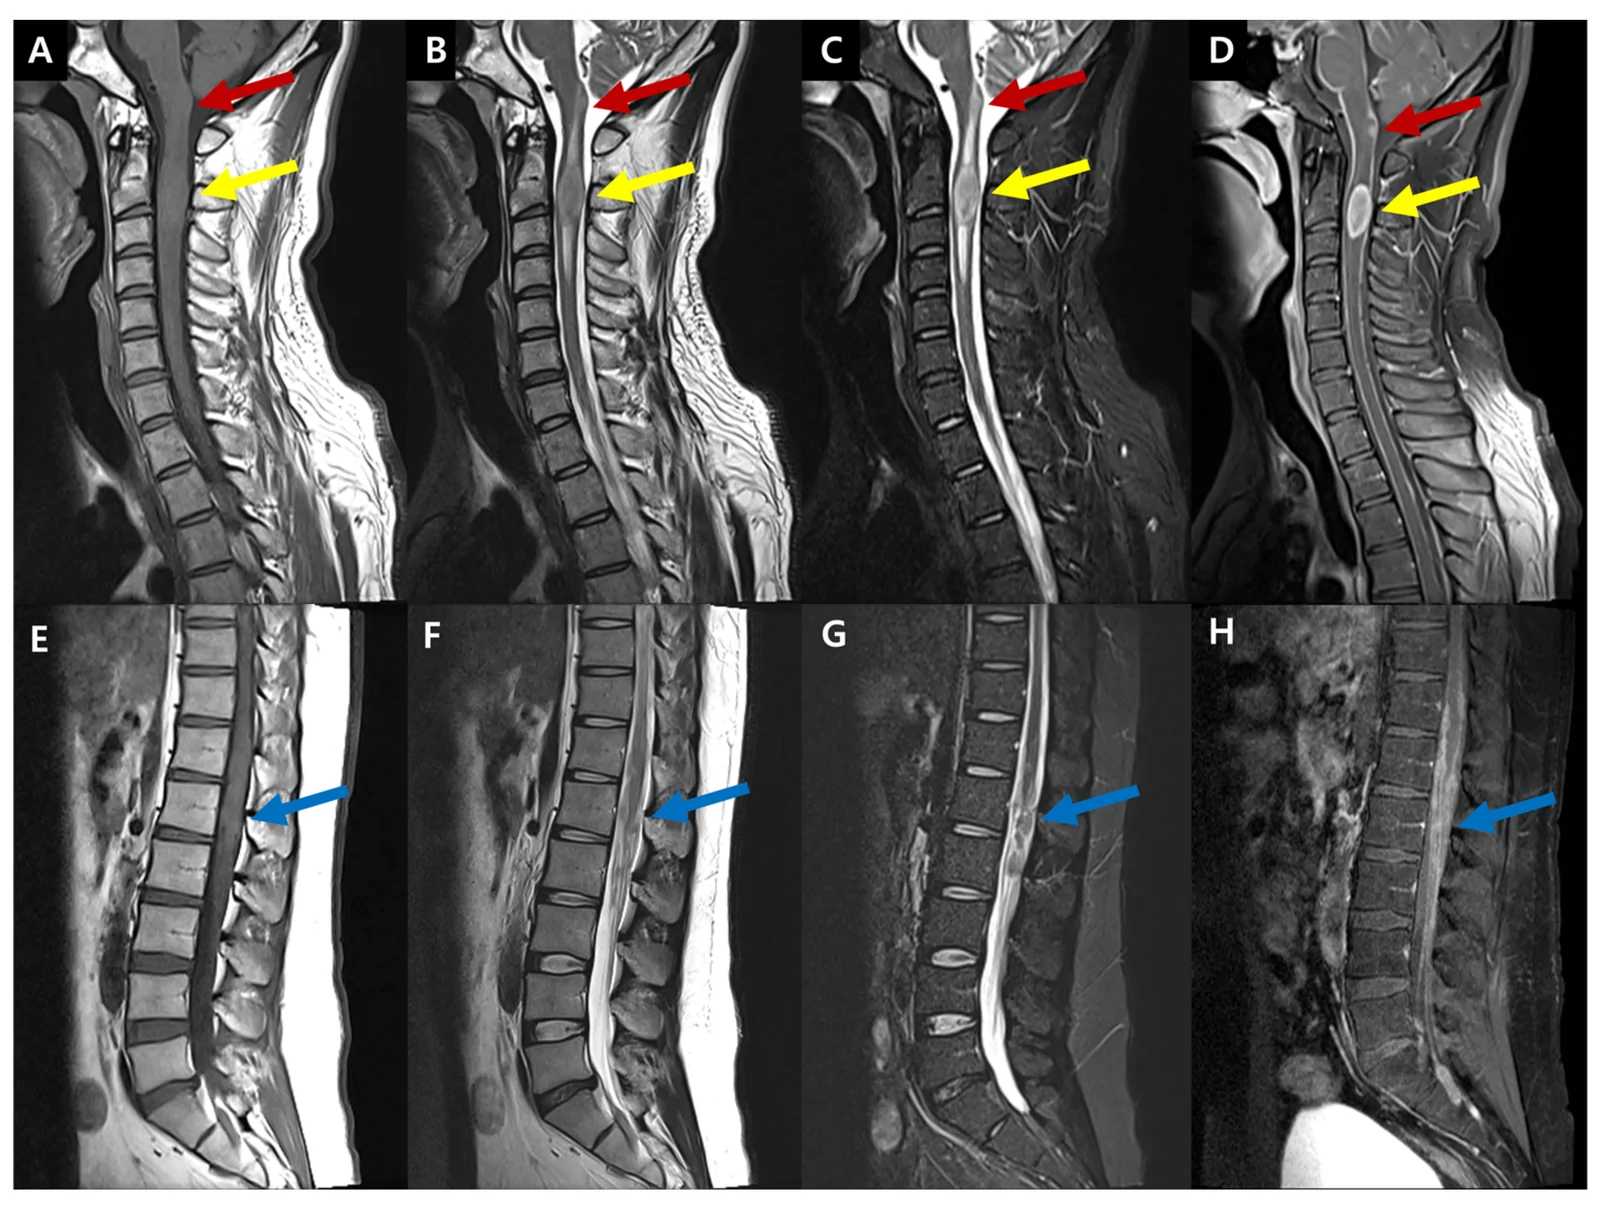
A 27-year-old man presented with motor weakness and radiating pain throughout the body. He had no history of hematologic malignancy, and routine laboratory evaluation was unremarkable. Brain, cervical spine, and lumbar spine MRI examinations were performed. Sagittal T1-weighted (A), T2-weighted (B), and STIR (C) images of the cervical spine revealed an expansile intramedullary lesion at the C2–3 level (yellow arrows) associated with spinal cord swelling and syringomyelia. The lesion was relatively well-circumscribed, appearing isointense on T1-weighted imaging (A), mildly hyperintense on T2-weighted imaging (B), and hyperintense on STIR imaging (C). Contrast-enhanced sagittal T1-weighted imaging (D) demonstrated homogeneous enhancement of the intramedullary lesion (yellow arrows). An additional enhancing lesion at the C1 level (red arrows) suggested disseminated disease. Sagittal T1-weighted (E), T2-weighted (F), and STIR (G) images of the lumbar spine demonstrated diffuse leptomeningeal dissemination along the lower spinal cord and cauda equina (blue arrows). Sagittal contrast-enhanced T1-weighted imaging (H) exhibited linear and nodular enhancement along the lower spinal cord and cauda equina (blue arrows). Brain MRI additionally demonstrated diffuse leptomeningeal enhancement in the posterior cranial fossa. Initial staging was performed using contrast-enhanced whole-body CT, brain MRI, and whole-spine MRI. Contrast-enhanced whole-body CT demonstrated no extracranial involvement, while MRI confirmed disease confined to the central nervous system, supporting the diagnosis of primary central nervous system histiocytic sarcoma. Routine laboratory investigations, including complete blood counts and peripheral blood smear examination, were unremarkable. Bone marrow biopsy was not performed due to the absence of clinical or laboratory findings suggestive of systemic hematologic involvement.
